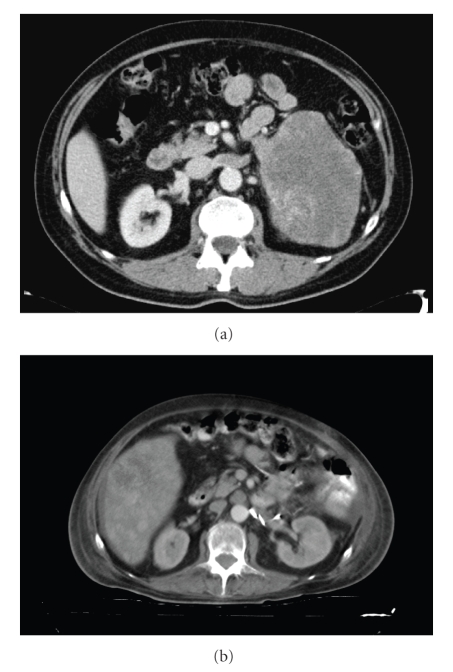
Pre- (a) and postoperative (b) CT scan showing tumour thrombosis of the left renal vein and later vascular result.
